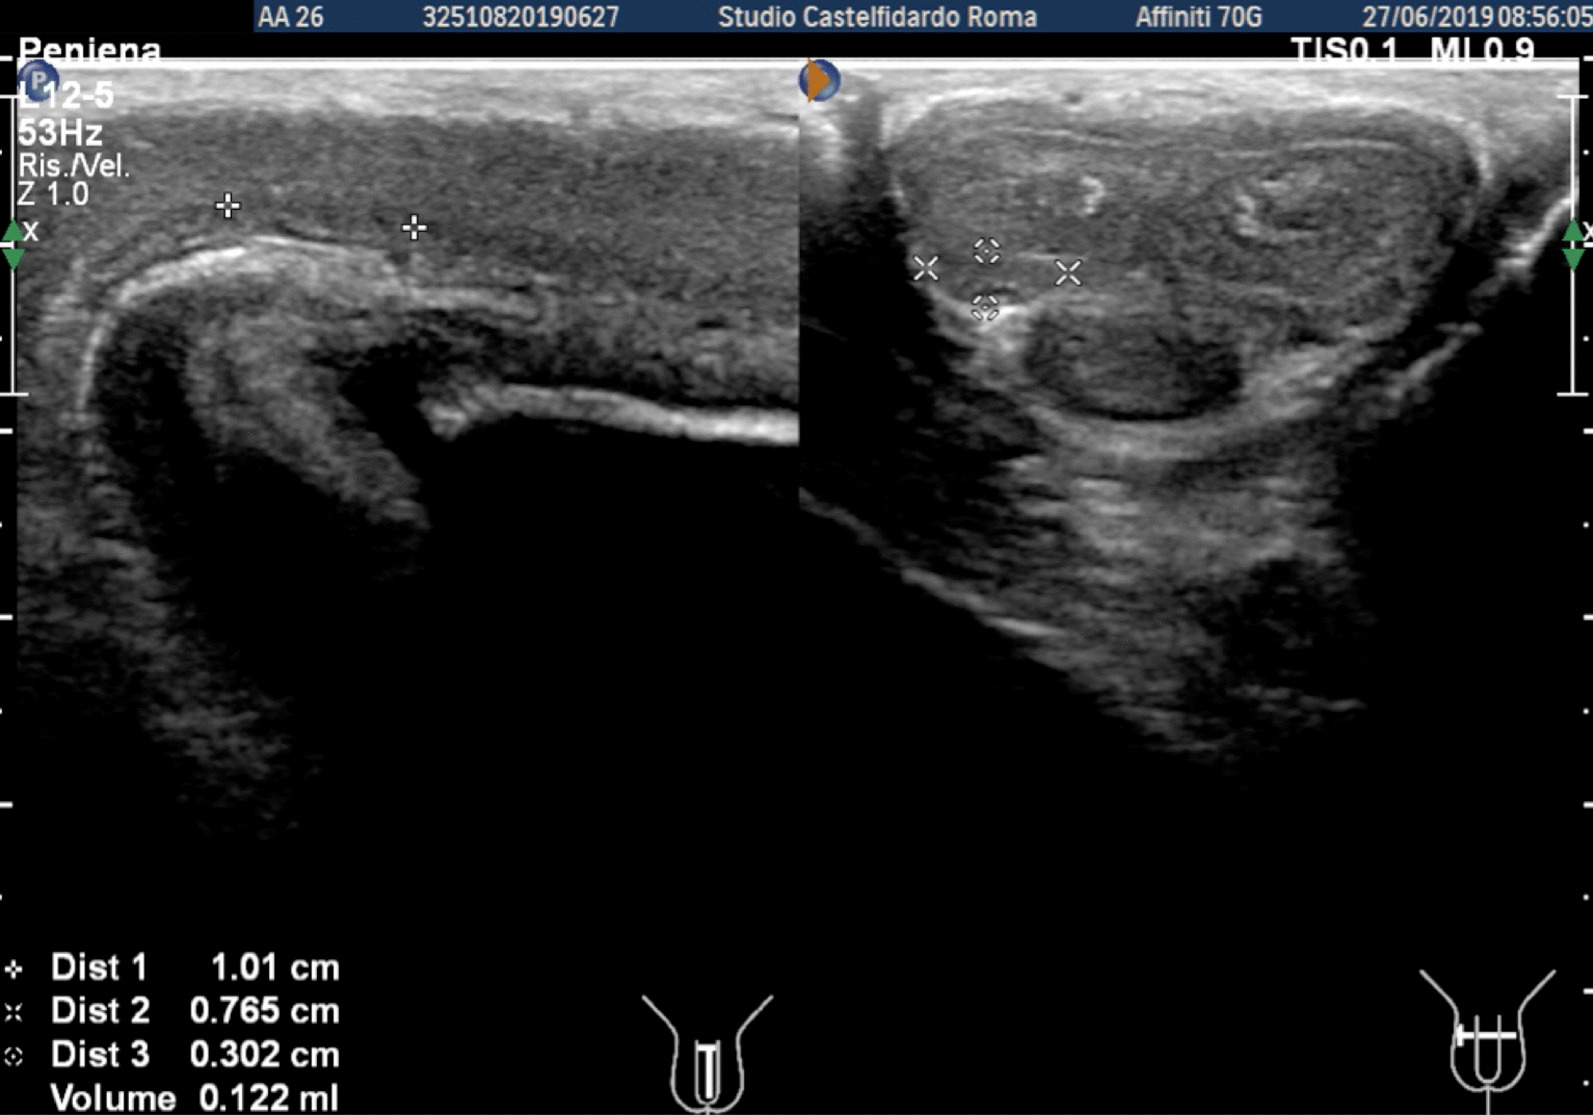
Penile ultrasound and plaque measurement prior to treatment (longitudinal and transverse scan).
